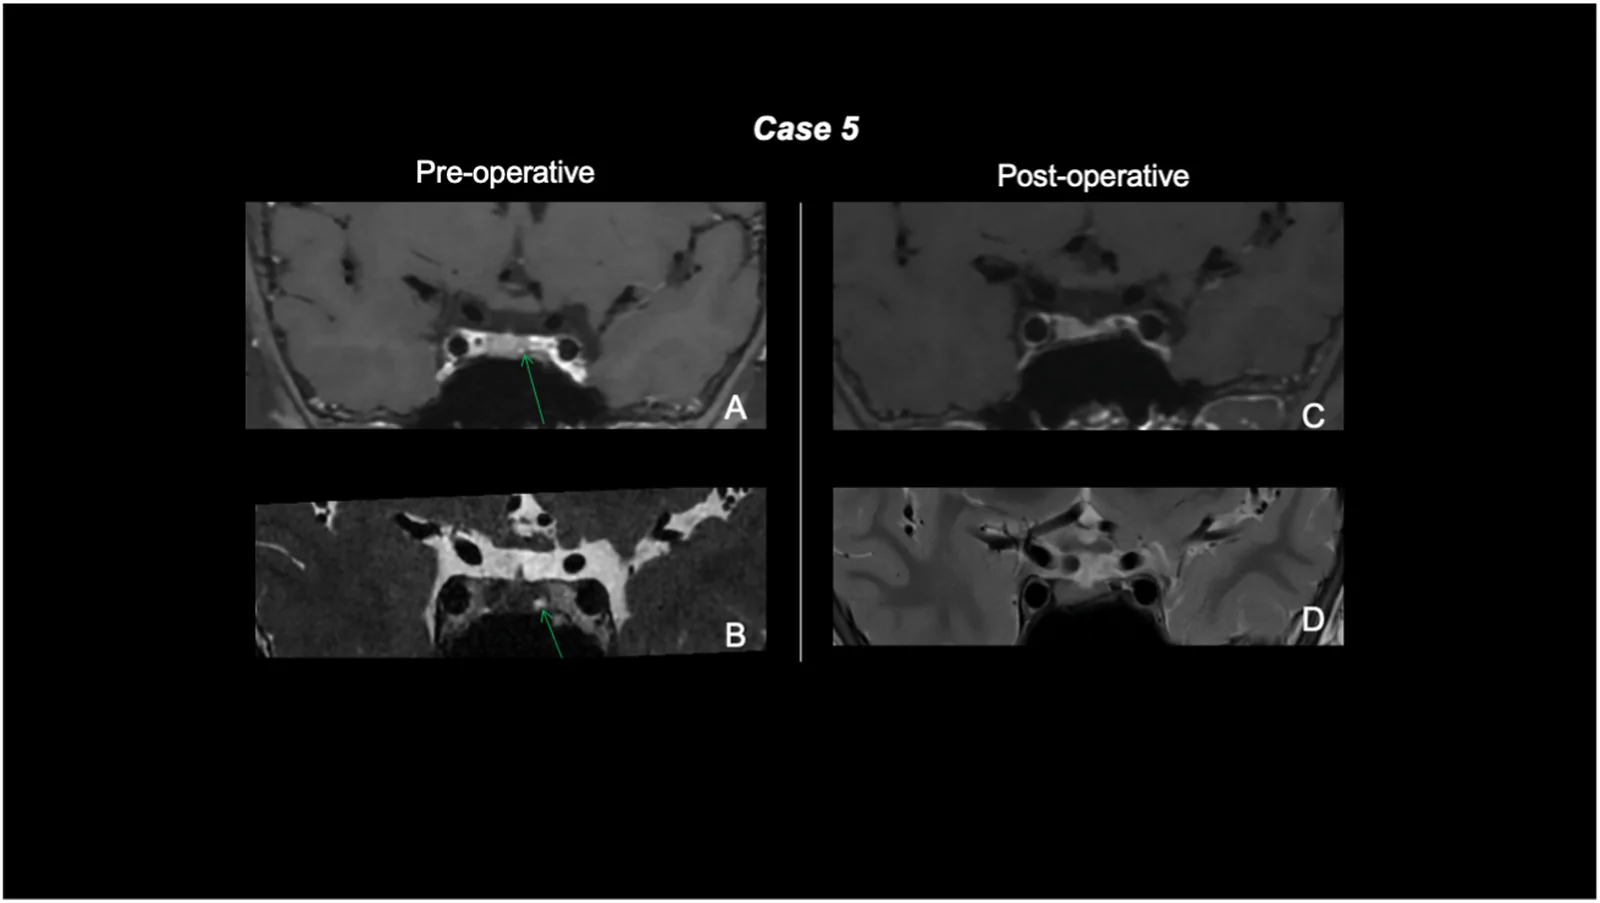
MRI-equivocal with a unifocal heterogeneous signal (Case 5) (A–B) Preoperative MRI (left) showing a 2-mm focal lesion in the left pituitary gland, characterized by spontaneous T1 and T2 hyperintensity (green arrows). Targeted surgical exploration of this heterogeneous signal was decided after multidisciplinary discussion. A tumor was identified and an enlarged adenomectomy was performed, resulting in endocrinological remission. (C–D) Postoperative MRI at 12 months showing no residual tumor.
